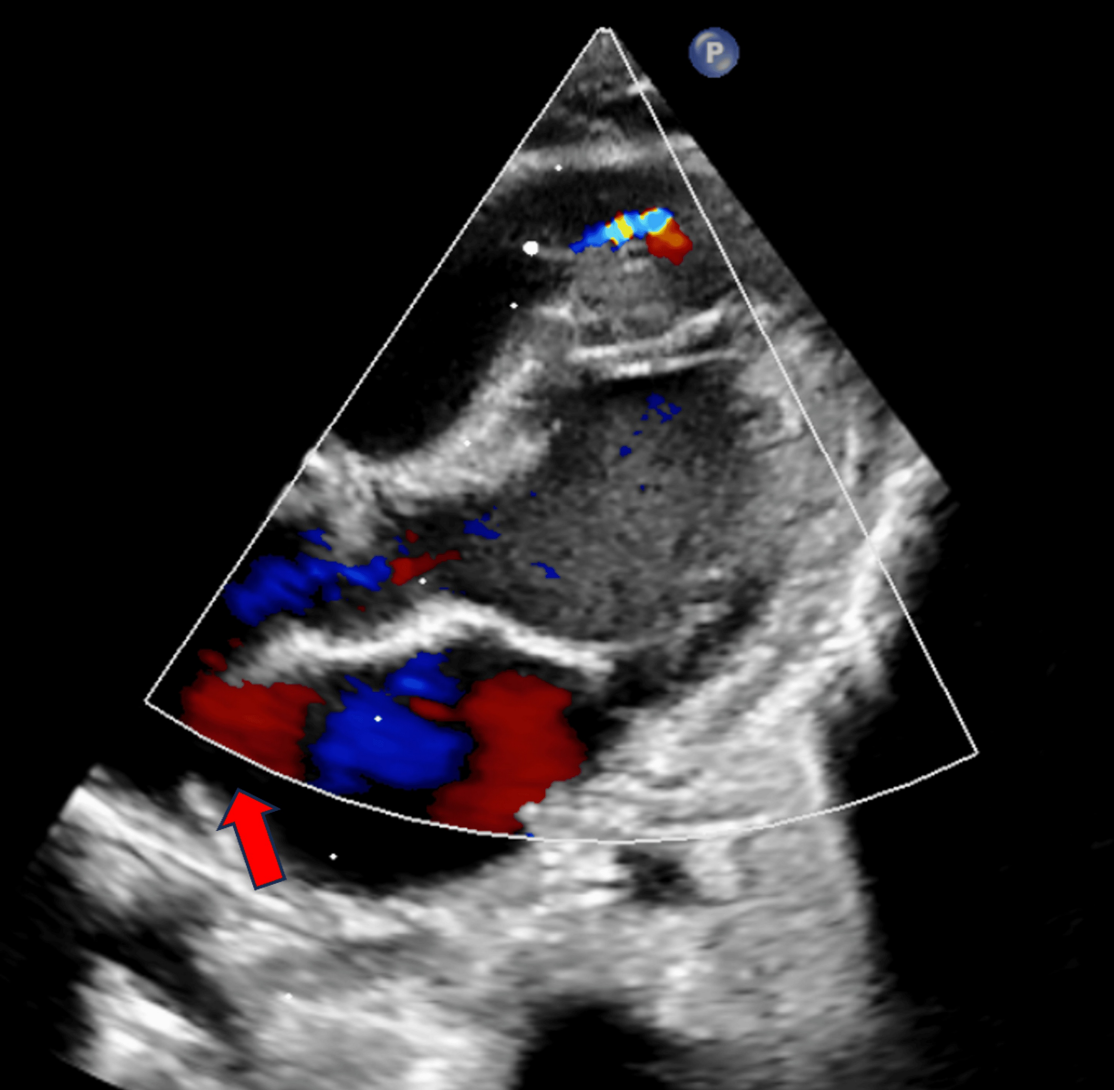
2D echocardiography indicating a ventricular septal defect (red arrow)
